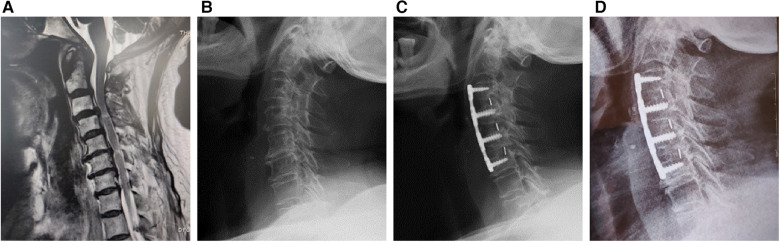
Male, 69-year-old, underwent three-level anterior cervical discectomy and fusion (ACDF) surgery. (A) The pre-operational magnetic resonance imaging (MRI), (B) the x-ray of pre-operation, (C) operation after 3 months, and (D) the final follow-up.
